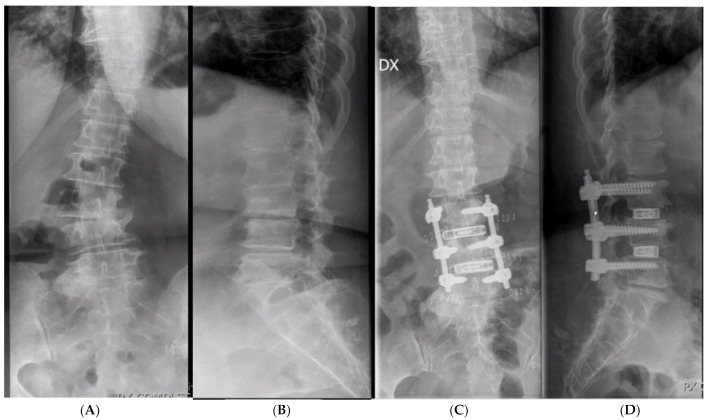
A 72-year-old women. (A,B) L3-L4 and L4-L5 DDD with right convex scoliosis and segmental L3-L5 kyphosis. (C,D) Postop X-rays showing L3-L4 and L4-L5 expandable OLIF cages supported by posterior instrumentation, with correction of the coronal plane. Note that expanded cages reach both lateral aspects of the epiphyseal ring with indirect foraminal decompression.
